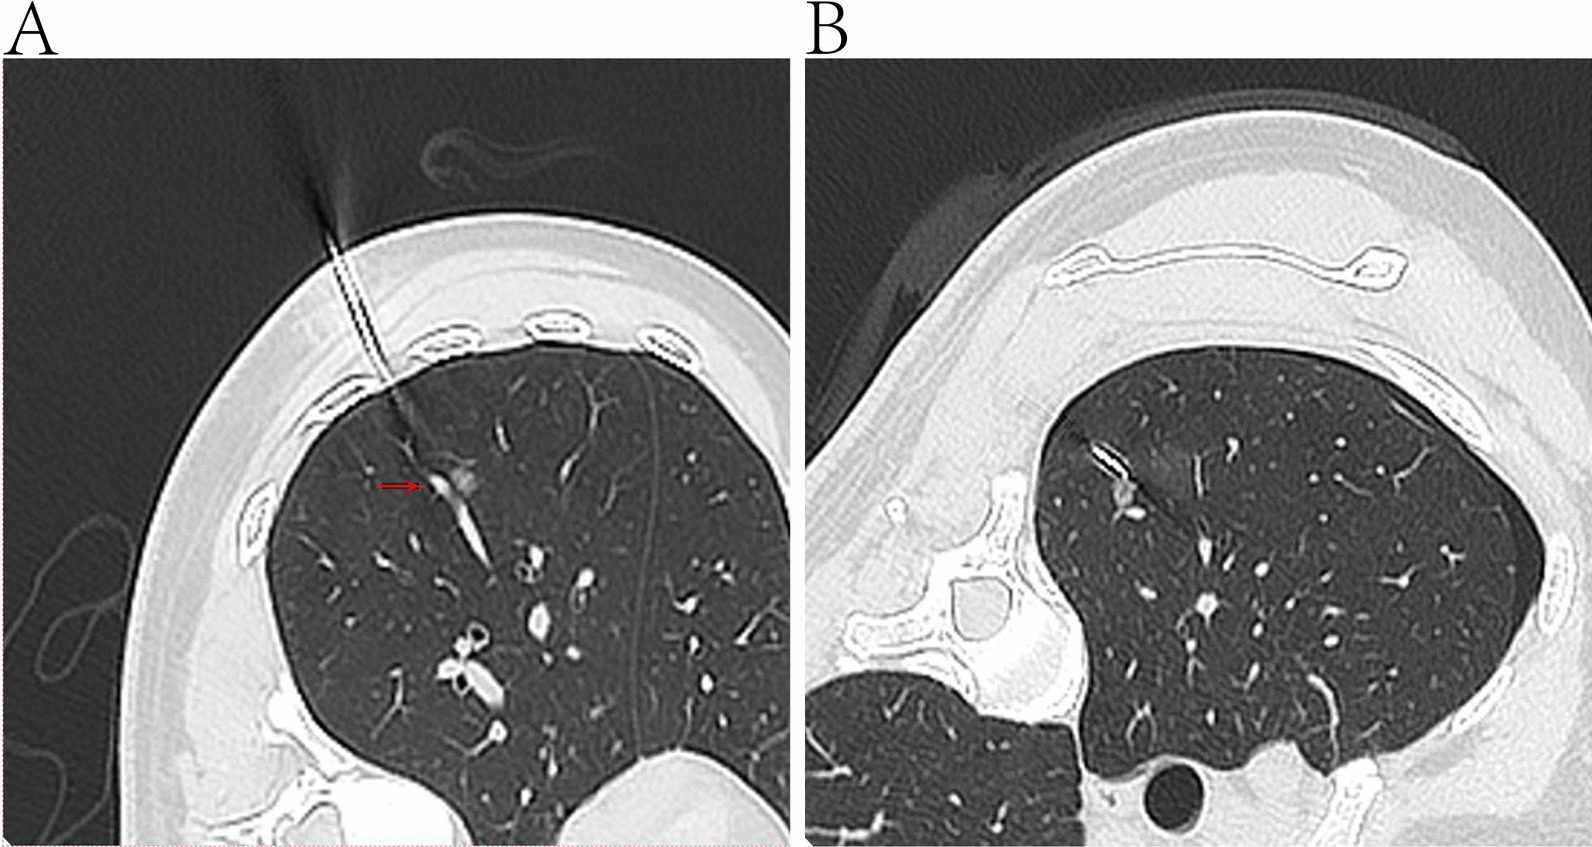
CT guided localization may be performed 30 min before the operation in order to achieve accurate resection according to the needs of the operation
